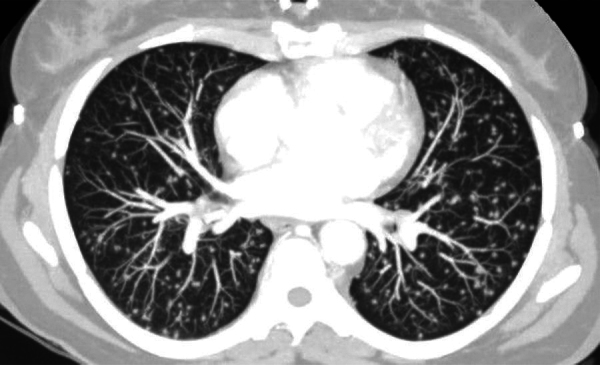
Computed tomography parenchymal window of the lung
